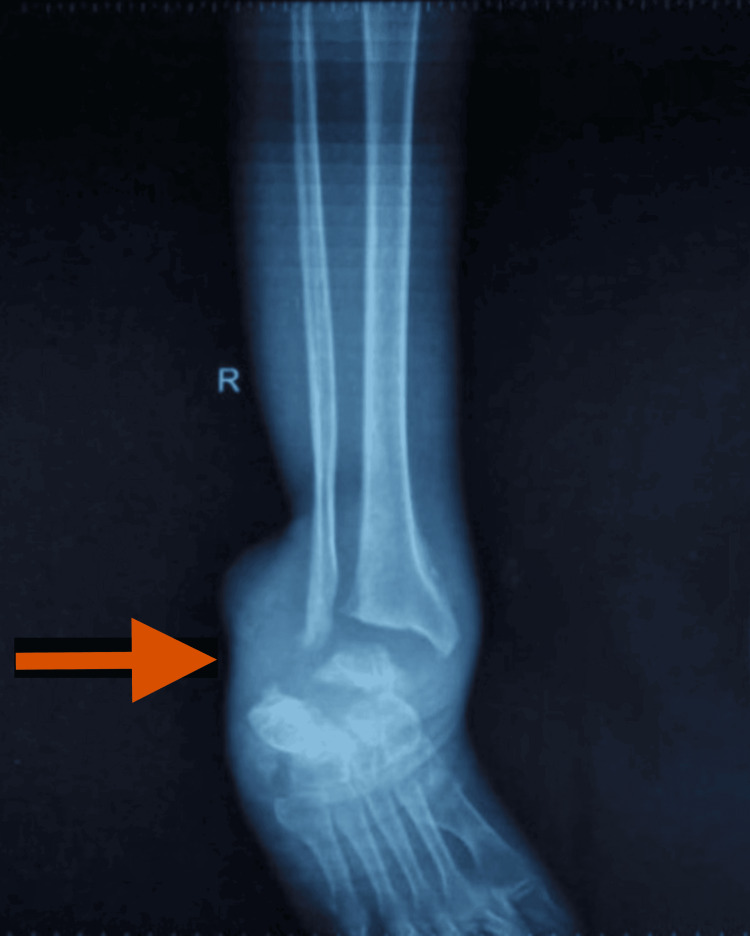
The radiograph shows bony destruction and deformity (arrow).
